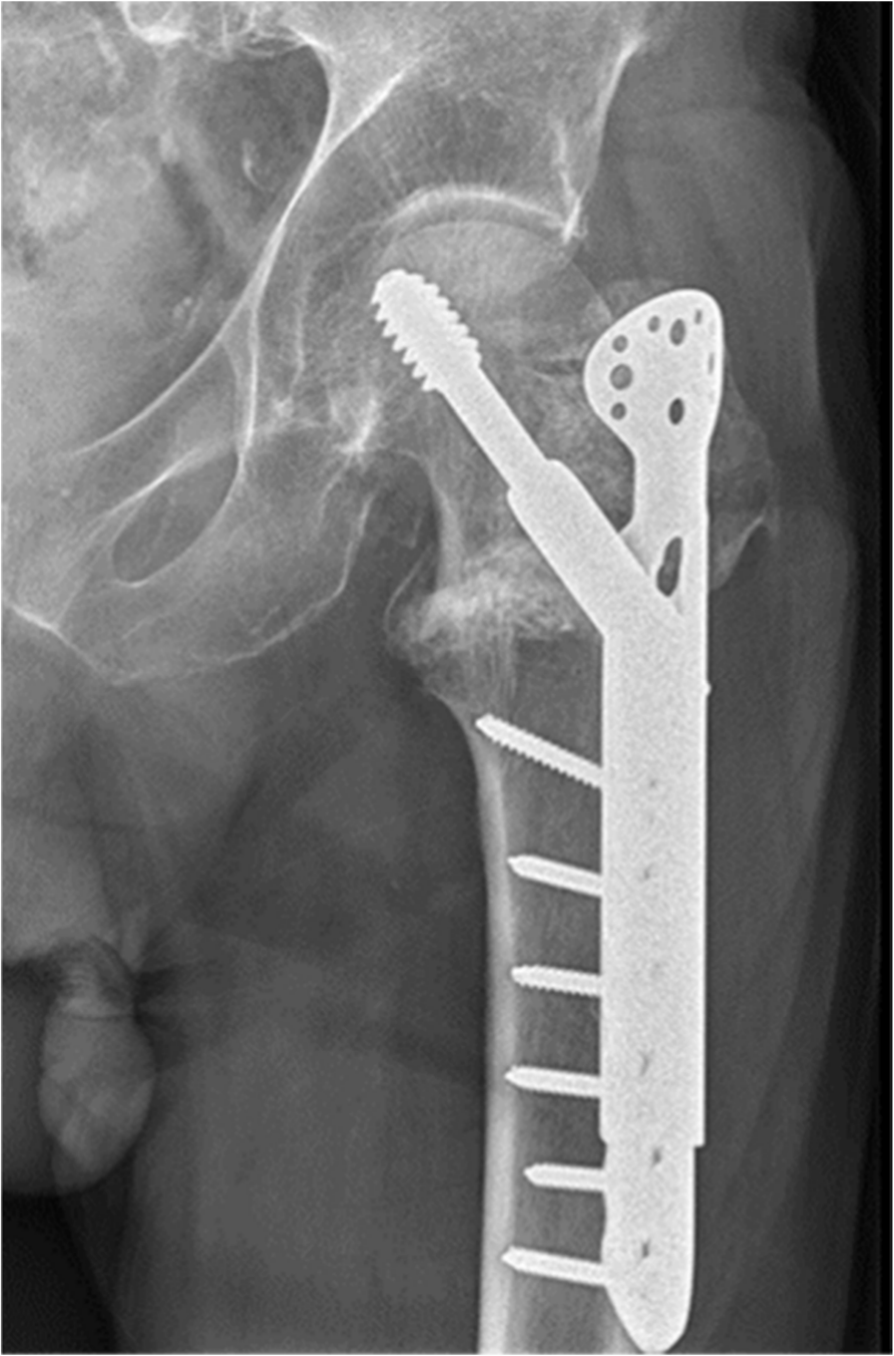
Radiograph of sliding hip screw with a modular trochanteric stabilizing plate in an AO/OTA 31A2 fracture (published with patient consent)
